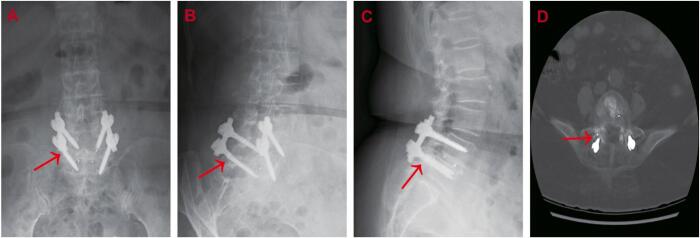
A-D. Postoperative anteroposterior (A), oblique (B) and lateral (C) radiographs and CT images (D) revealed the fracture of a screw (arrow).
